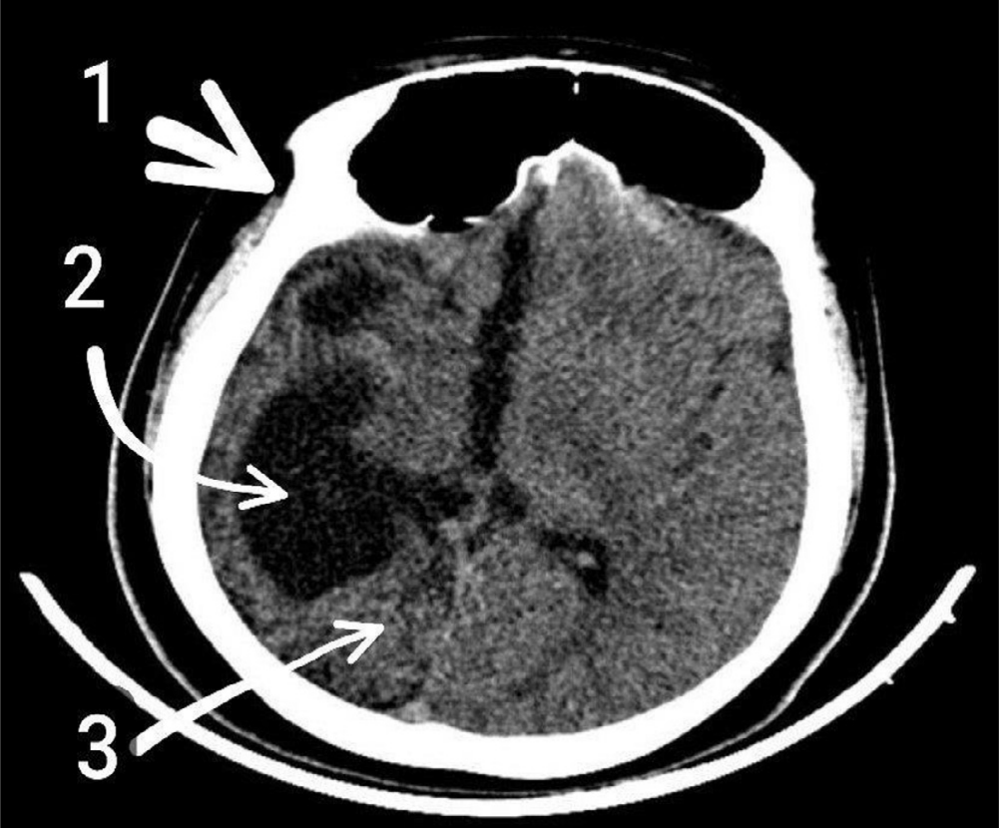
(CT brain—soft tissue window): Noncontrast axial CT scan of the brain in soft tissue window demonstrates marked atrophy of the right cerebral hemisphere with associated ipsilateral ventricular dilatation (ventriculomegaly) and compensatory thickening of the calvarium on the same side. Although optimal visualization of bony structures such as mastoid air cells is limited in the soft tissue window, suggestive features of right-sided mastoid hyperpneumatization can still be appreciated. 1. Calvarial thickening. 2. Ipsilateral ventriculomegaly. 3. Right cerebral hemisphere atrophy.
